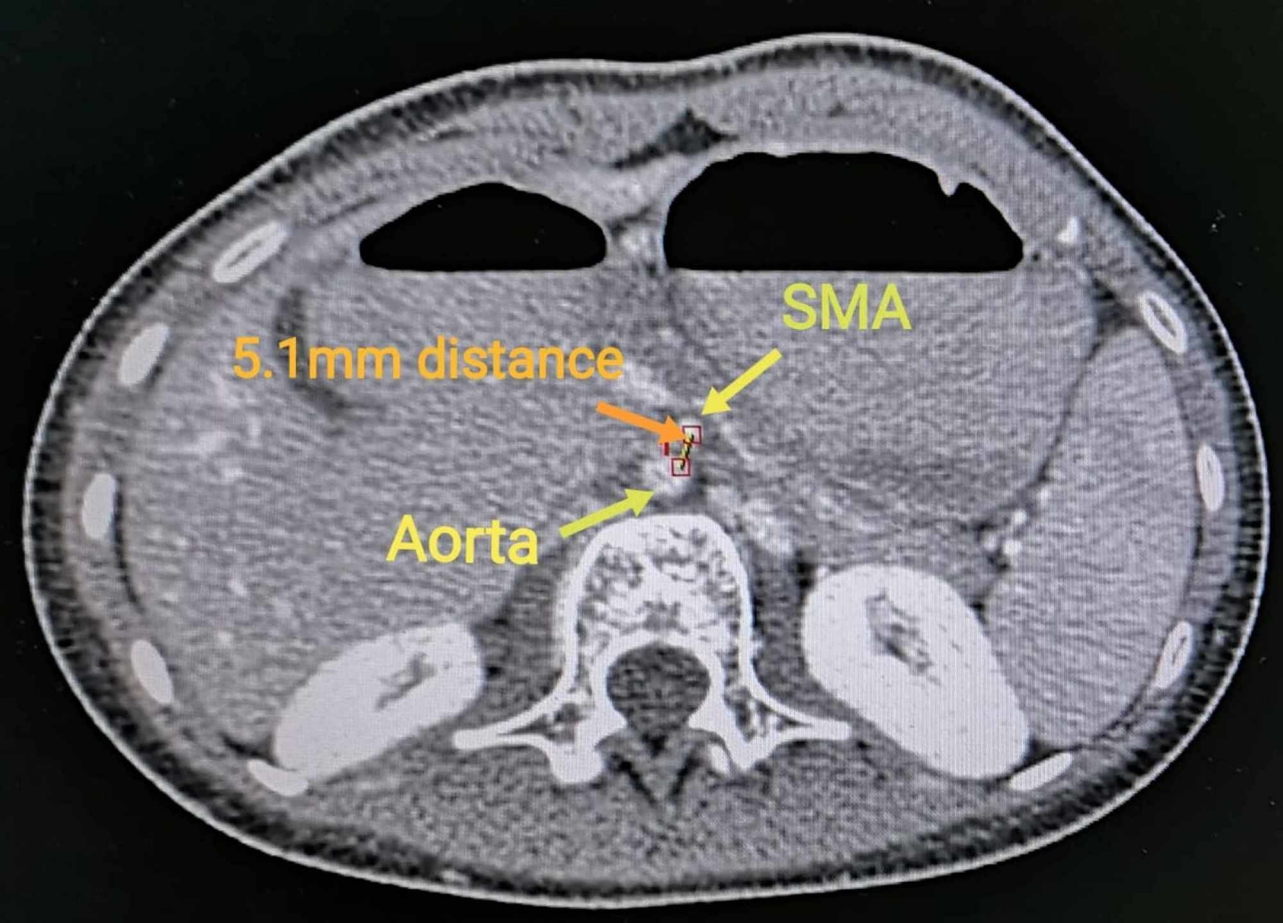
CT scan showing a reduced distance of 5.1 mm (normal 10-20 mm) between SMA and aorta, insinuating a diagnosis of superior mesenteric artery syndrome. SMA: superior mesenteric artery
